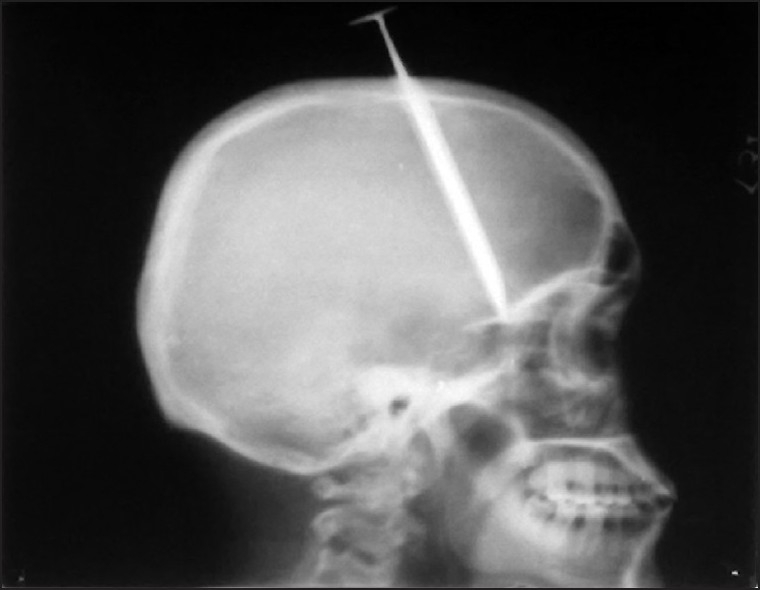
X-ray skull lateral view showing nail depth
